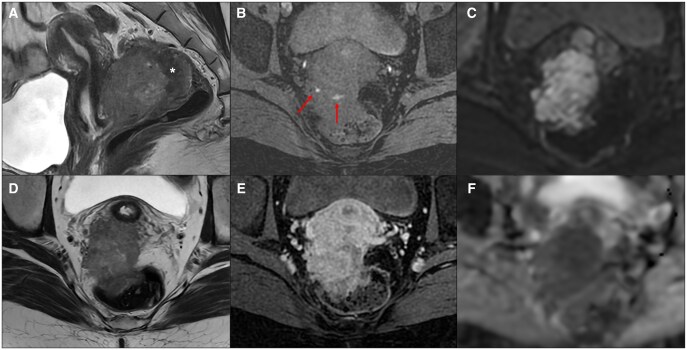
Decidualized DPE in the posterior cul-de-sac. Pelvic MRI demonstrates a solid mass centered in the posterior cul-de-sac, infiltrating the cervix and the rectum. On sagittal and axial T2-weighted images (A and D), the lesion shows intermediate signal intensity, slightly hyperintense relative to the pelvic musculature. The typical *mushroom cap* sign of bowel endometriosis is also visible on the sagittal T2WI (A, star). Fat-suppressed T1-weighted images demonstrate small intralesional hemorrhagic foci (B, arrows). Diffusion-weighted imaging and the corresponding ADC map show marked diffusion restriction (C and F), while post-contrast fat-suppressed T1-weighted images demonstrate avid enhancement of the solid component (E).
